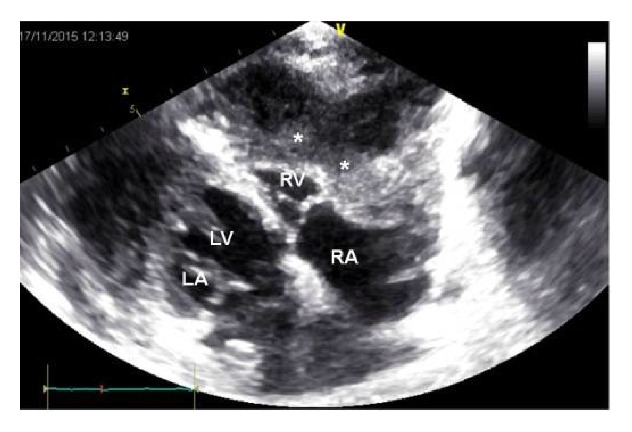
Echocardiography: Increased pericardial echogenity, pericardial thickening, localized liquid collections in apex adherent to pericardium (*∗*), and deviation of interventricular septum to left in inspiration (favoring pulmonary hypertension) and diastolic dysfunction due to constrictive pericarditis.
